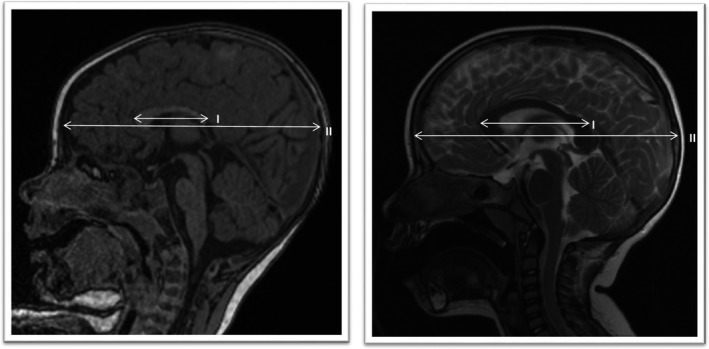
Midsagittal MRI images. Left: Patient 1. One‐year‐old boy with corpus callosum dysgenesis. T1‐FLAIR. Width I:II = 1:3.6. Right: Normal control. Two‐year‐old boy. T2‐FLAIR. Width I:II = 1:2.2. Case courtesy of *Dr Bruno Di Muzio*, Radiopaedia.org
